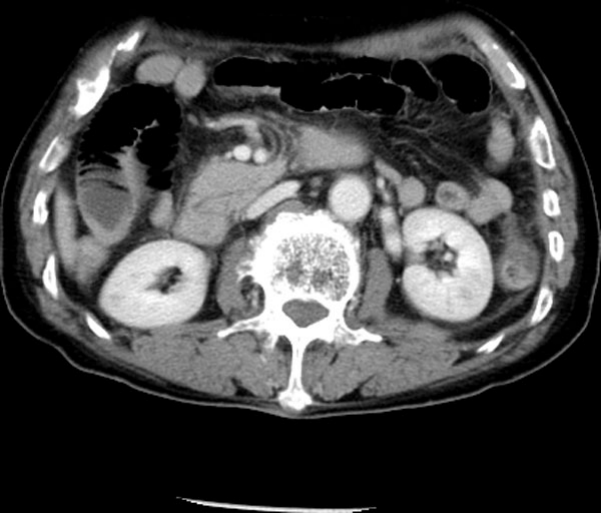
Axial CT scan obtained with IV contrast material in 76-year-old male subject diagnosed with ischemia.
